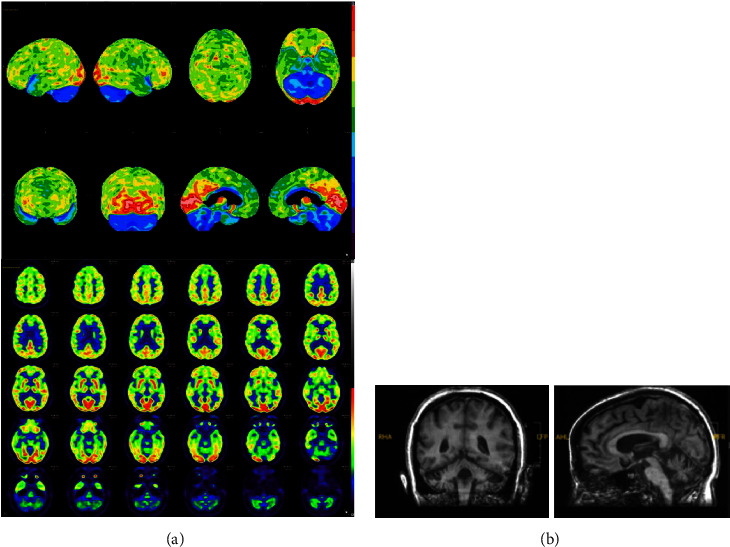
(a) Patient's FDG-PET scan at age 39 showed severe hypometabolism in the cerebellum, medial temporal lobes, paramedial frontal lobes, midbrain, and pons. There was also mild-to-moderate hypometabolism in most of the cerebral cortex except the bilateral occipital lobes, precuneus, and posterior cingulate. (b) Patient's MRI T1 sagittal and coronal scan at age 39 showed cerebellum atrophy.
